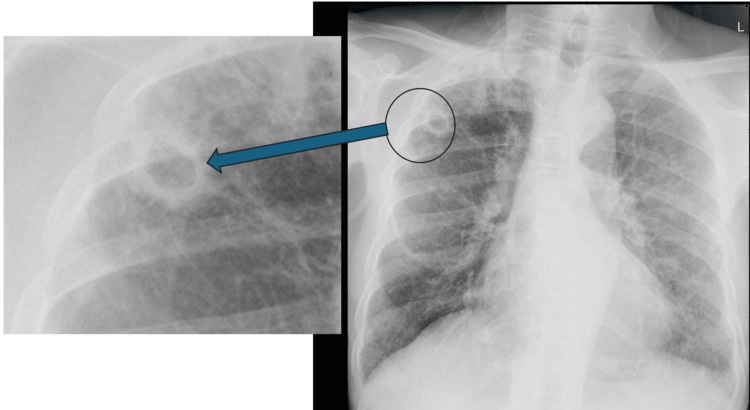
Postero-anterior chest x-ray image of a 65-year-old male patient with more than two weeks history of cough shows a right upper zone thick-walled cavity (blue arrow), indicative of active tuberculosis. Image Credit: Field data from the 2013 National TB prevalence survey. Permission was obtained from Dr. Frank Adae Bonsu, the Principal Investigator and the original data custodian of the 2013 National TB Prevalence Survey, to use the image.
